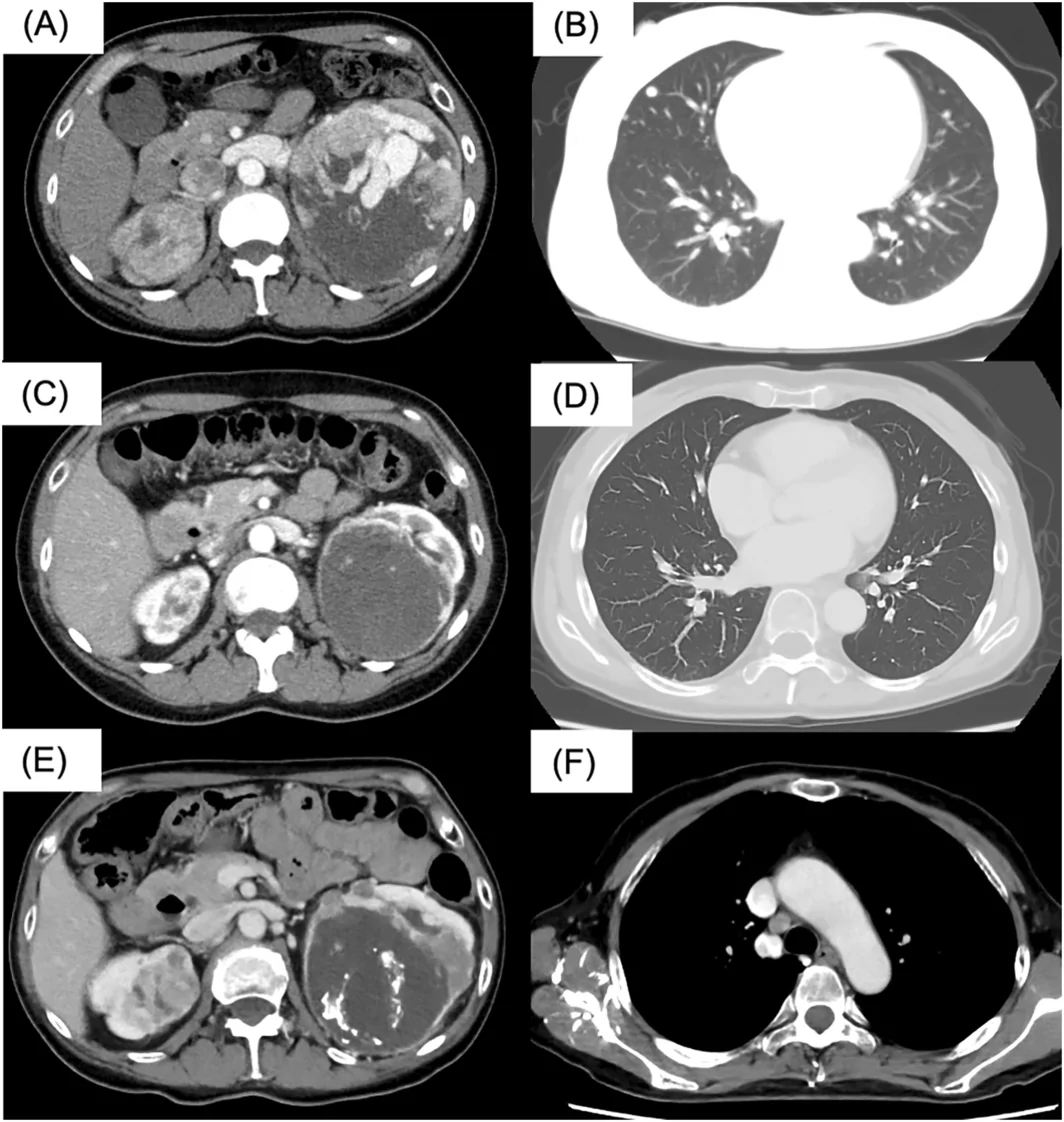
Serial contrast‐enhanced computed tomography findings during systemic therapy. (A, B) At initial diagnosis, contrast‐enhanced computed tomography demonstrated bilateral renal tumors (A) and pulmonary metastases (B). Pancreatic metastasis was also identified but is not shown. The disease was staged as cT3aN0M1. (C, D) After prior systemic therapy, the pulmonary and pancreatic metastases had achieved complete response, whereas the bilateral renal tumors remained. (E, F) Immediately before initiation of belzutifan, the bilateral renal tumors showed disease progression (E), and new mediastinal and right hilar lymph node metastases were identified (F), leading to initiation of belzutifan as third‐line therapy.
